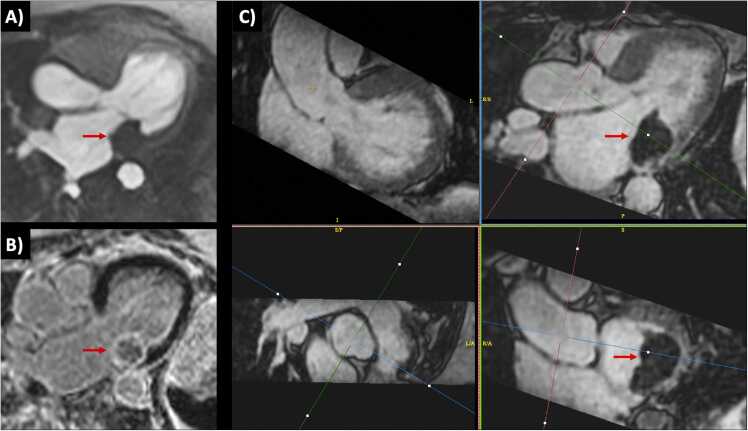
Patient with left atrial mass (arrows) discovered on echocardiography, likely caseous mitral annular calcification. (A) The lesion appears dark with first-pass perfusion imaging. (B) Late gadolinium enhancement image using single-shot inversion-recovery bSSFP shows mild peripheral enhancement. (C) The lesion is well depicted in multiplanar reconstructions from equilibrium phase bT1RESS. *bT1RESS* balanced T1 relaxation-enhanced steady-state, *bSSFP* balanced steady-state free precession.
